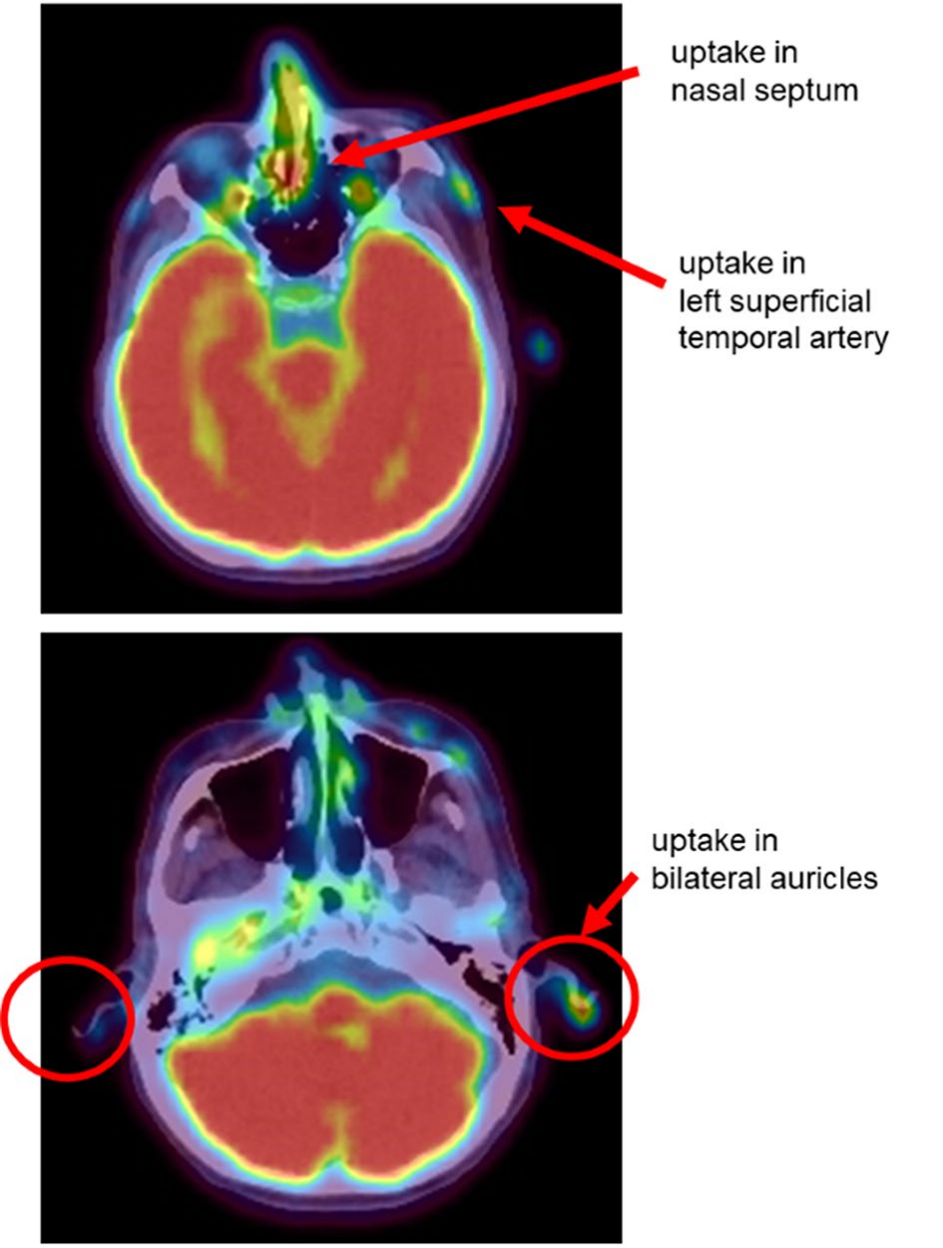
^18^F-FDG PET/CT images of the patient’s head. ^18^F-FDG PET/CT images demonstrated intense FDG uptake in his left superficial temporal artery, nasal septum, and bilateral auricles
